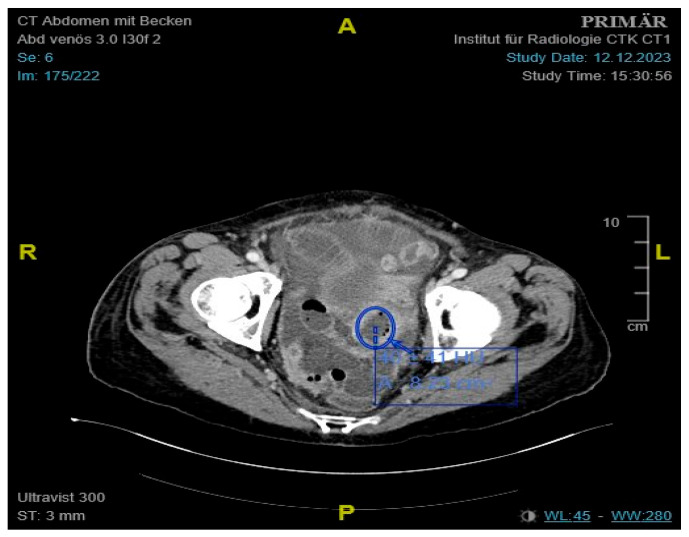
Contrast-enhanced CT of the abdomen showing an approximately 4.1 cm mass, consistent with a small bowel conglomerate tumor.
